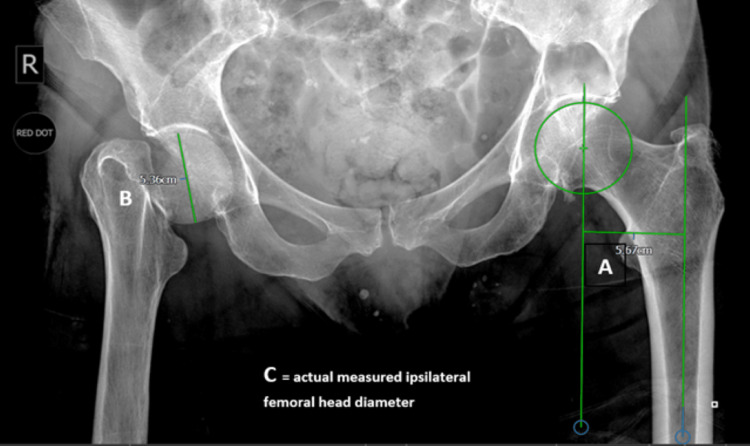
Preoperative radiograph with measurements
